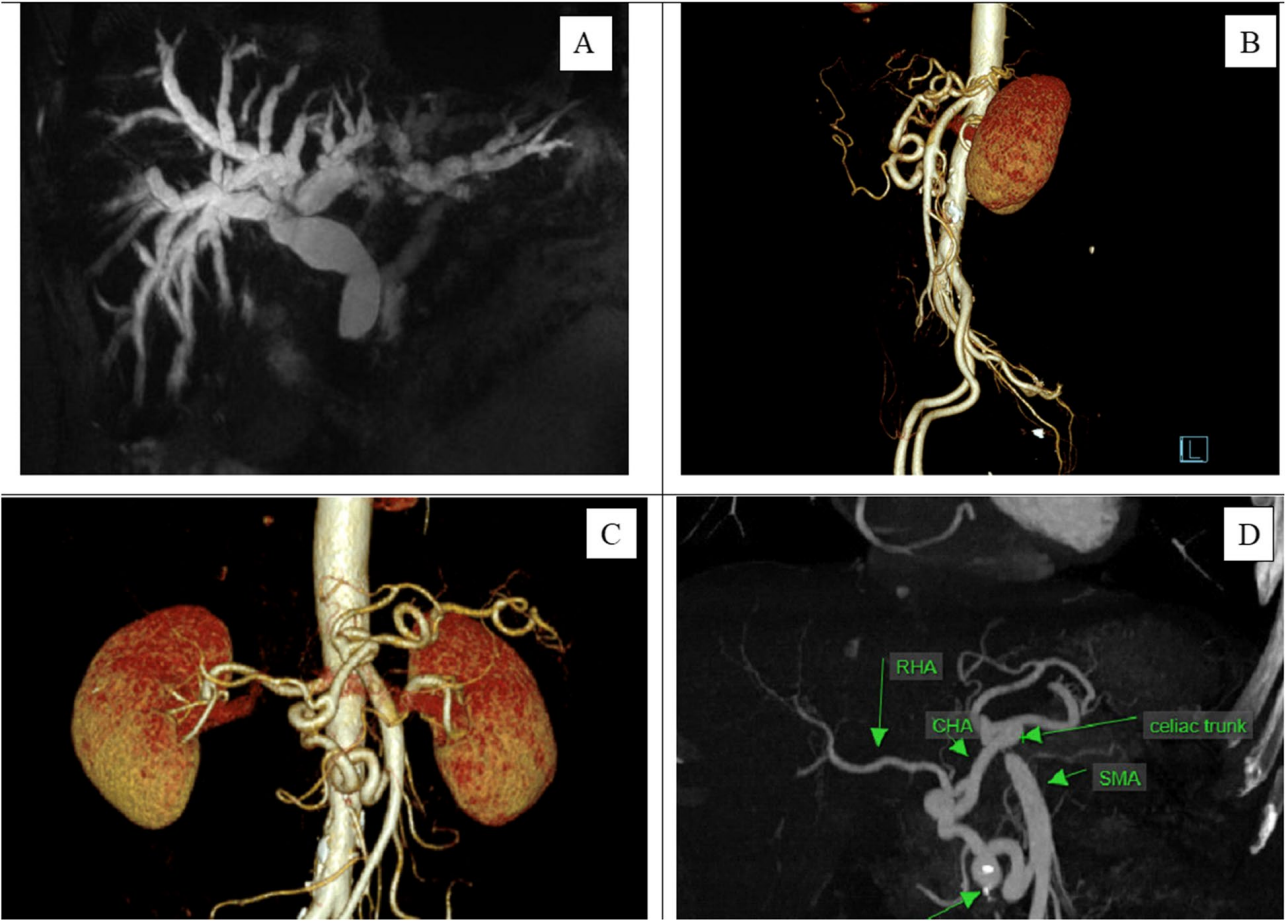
Gadoxetic acid-enhanced magnetic resonance imaging revealed a strict distal common bile duct with enhancement in the early phase (A). Three-dimensional reconstruction images showed developed collateral pathways around the pancreatic head (B, C). In the sagittal view, the CA was compressed severely by the MAL, which developed caudally (D) (CT, celiac trunk; CHA, common hepatic artery; GDA, gastroduodenal artery; IPDA, inferior pancreaticoduodenal artery; SMA, superior mesenteric artery; RHA, right hepatic artery)
